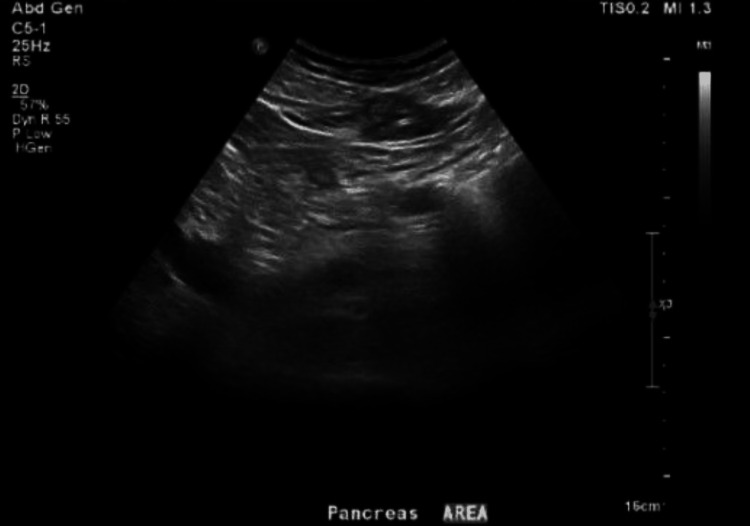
Abdominal ultrasound showed only fatty liver and the pancreas was obscured by bowel gas
